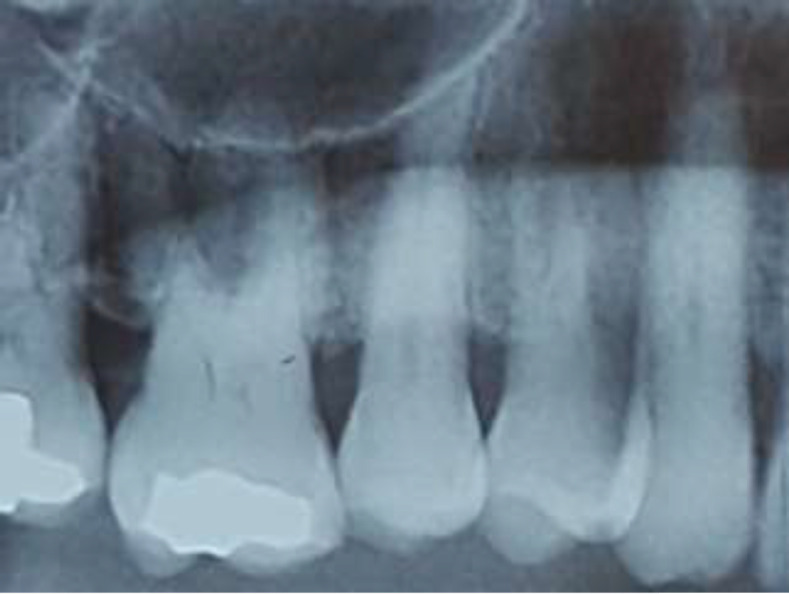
No osteolytic lesion on radiography
